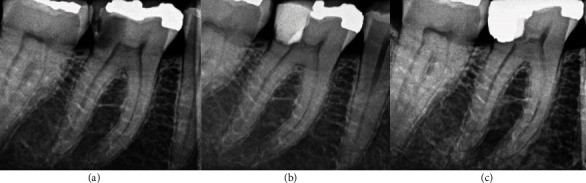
Permanent tooth 46. The image (a) shows diagnostic X-ray, image (b) immediate postoperative, and image (c) control at 24 months.
